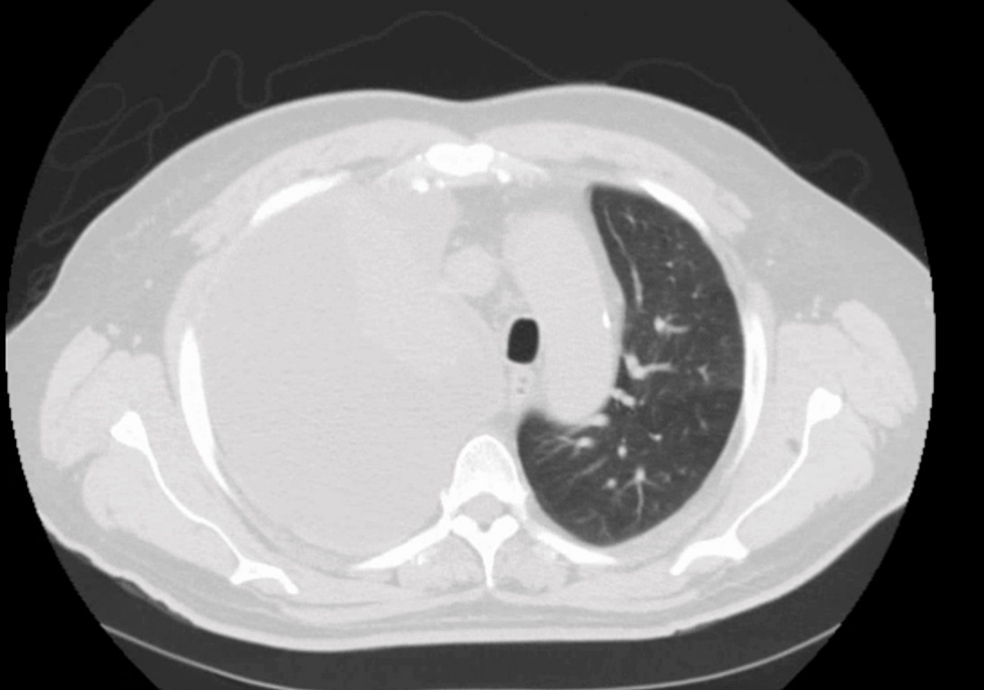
CT chest showing large right pleural effusion.
